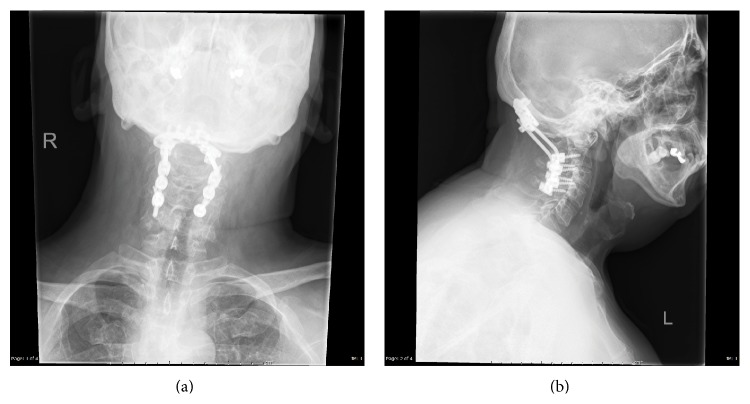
AP (a) and lateral (b) X-rays depicting an occipitocervical fusion.
